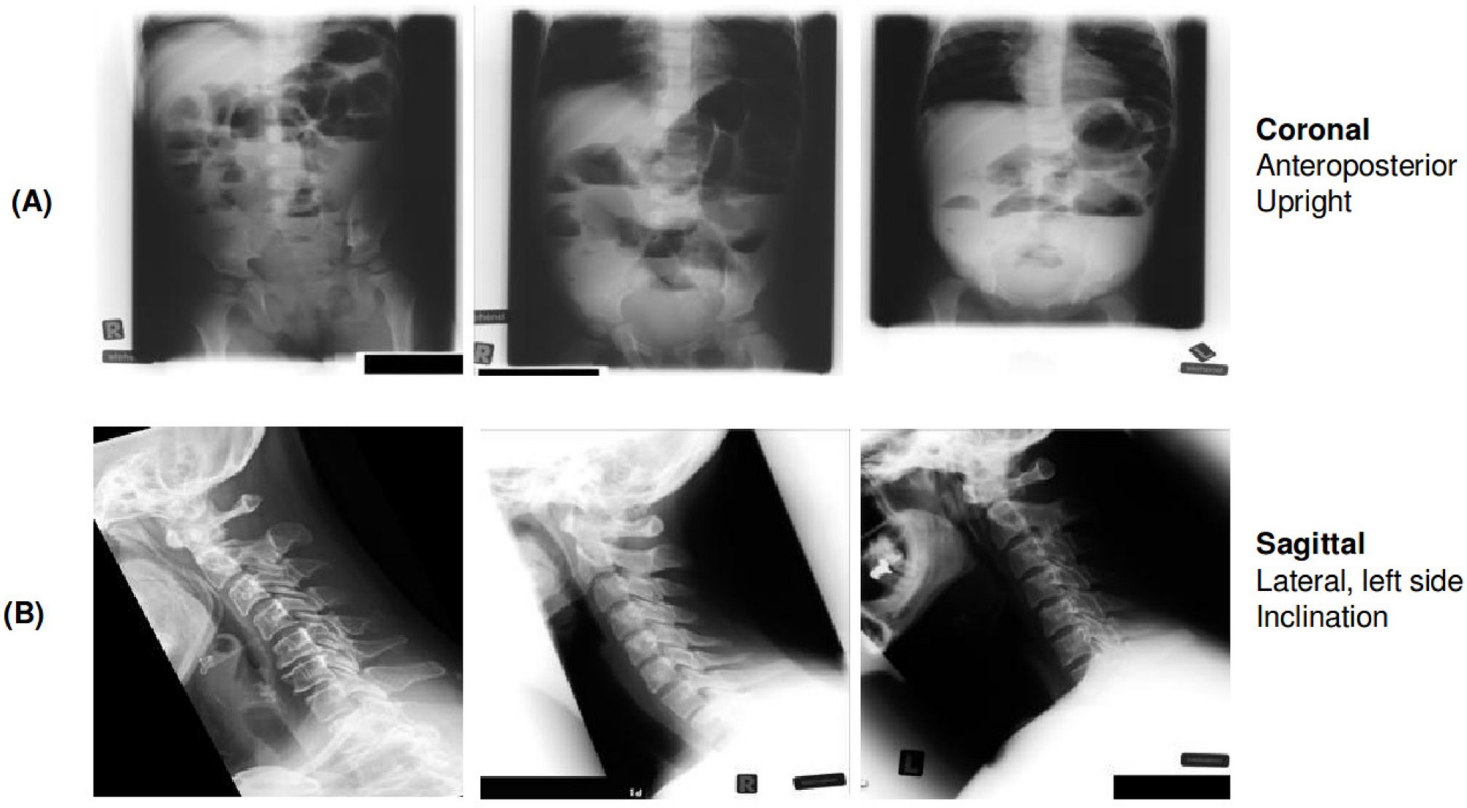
Examples of radiographs annotated with two classes from the D-scheme. (A) shows three image belonging to class ‘125’ representing ‘Coronal; Anteroposterior; Upright’ and (B) displays three images belonging to class ‘228’ representing ‘Sagital; Lateral, left-right; Inclination’. All radiographs were randomly chosen from the ImageCLEF 2009 Medical Annotation Task Training Set. Republished from [21] under a CC BY license, with permission from [RWTH Aachen], original copyright [2009].
